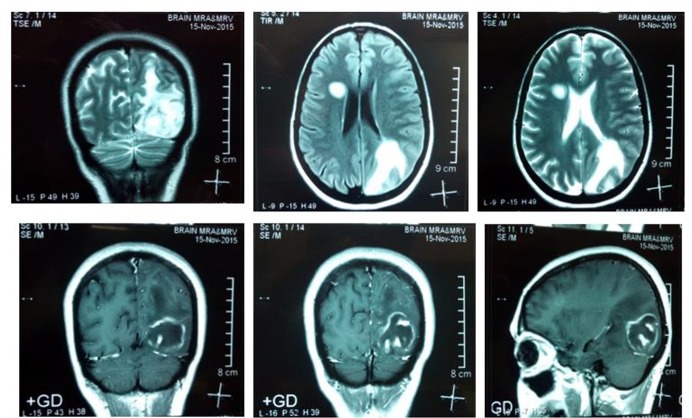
The T_1_-weighted MRI after gadolinium injection shows multiple rings enhancement lesions with surrounding edema in right temporal left parietal lobe of brain a patient with toxoplasmic encephalitis
